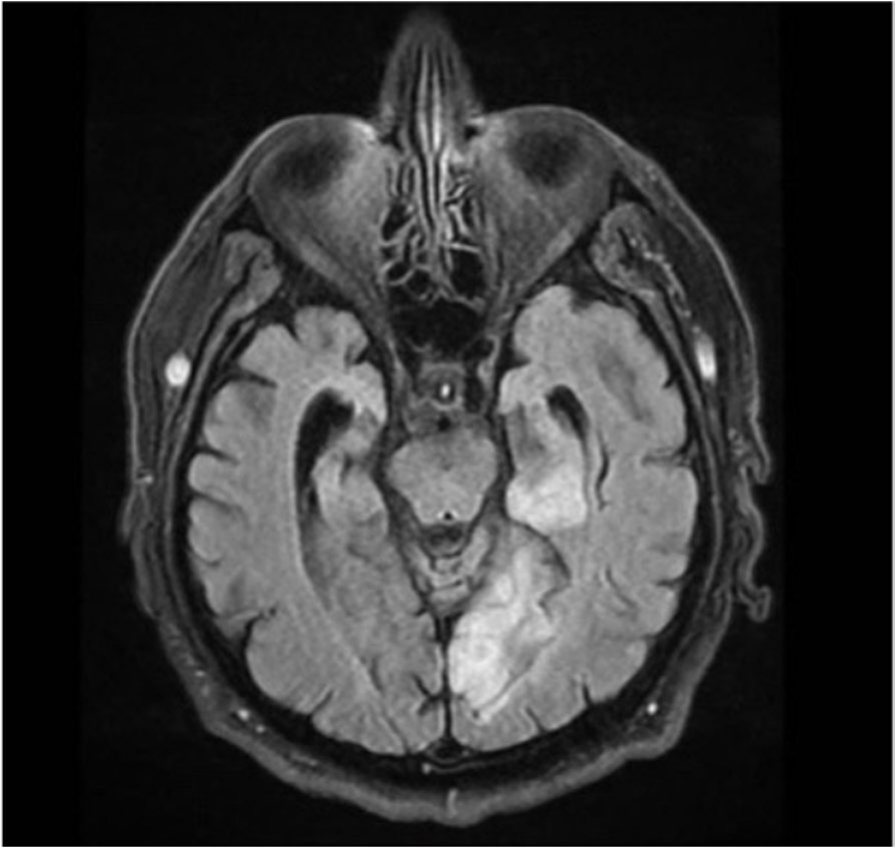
Left meso-temporal hyperintense signal in T2-weighted and FLAIR sequences
